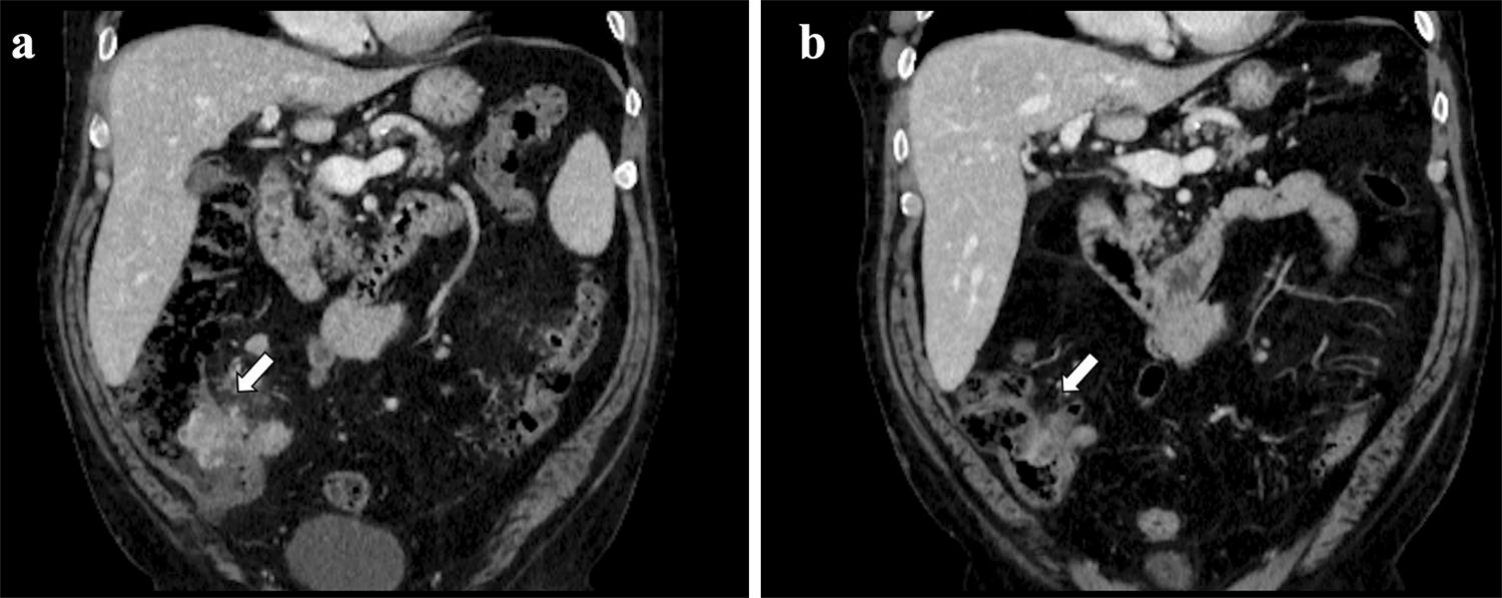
A 7-cm long contrast-enhanced marked thickening of the last ileal tract, along with the involvement of caecum and appendix, perivisceral adipose tissue suffusion and multiple enlarged lymph nodes at CT scan carried out 2 months after the beginning of BRAF/MEK TKIs (a); almost complete remission of previous radiological findings at CT scan performed after 1 month from treatment interruption (b)
